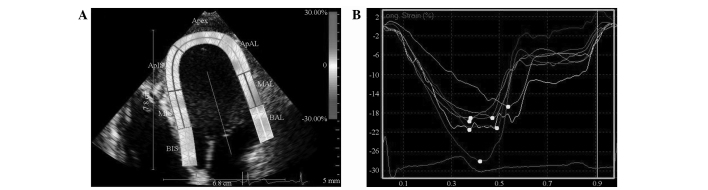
Apical four-chamber view STI measurement of left ventricular longitudinal strain and a strain-time curve. (A) Speckle-tracked overlay images of ROIs. The software was started and three points were selected. The software automatically traced the left ventricular wall, posterior septum and apical endocardium and epicardium, and equally divided the LV wall into the ventricular wall as basal, middle and apical segments. (B) Left ventricular lateral wall, posterior septum and apical strain-time curves. The average values of the basal, middle and apical segment of the corresponding ventricular wall were calculated to represent the strain values of the ventricular wall. STI, speckle-tracking imaging; ROI, region of interest.
